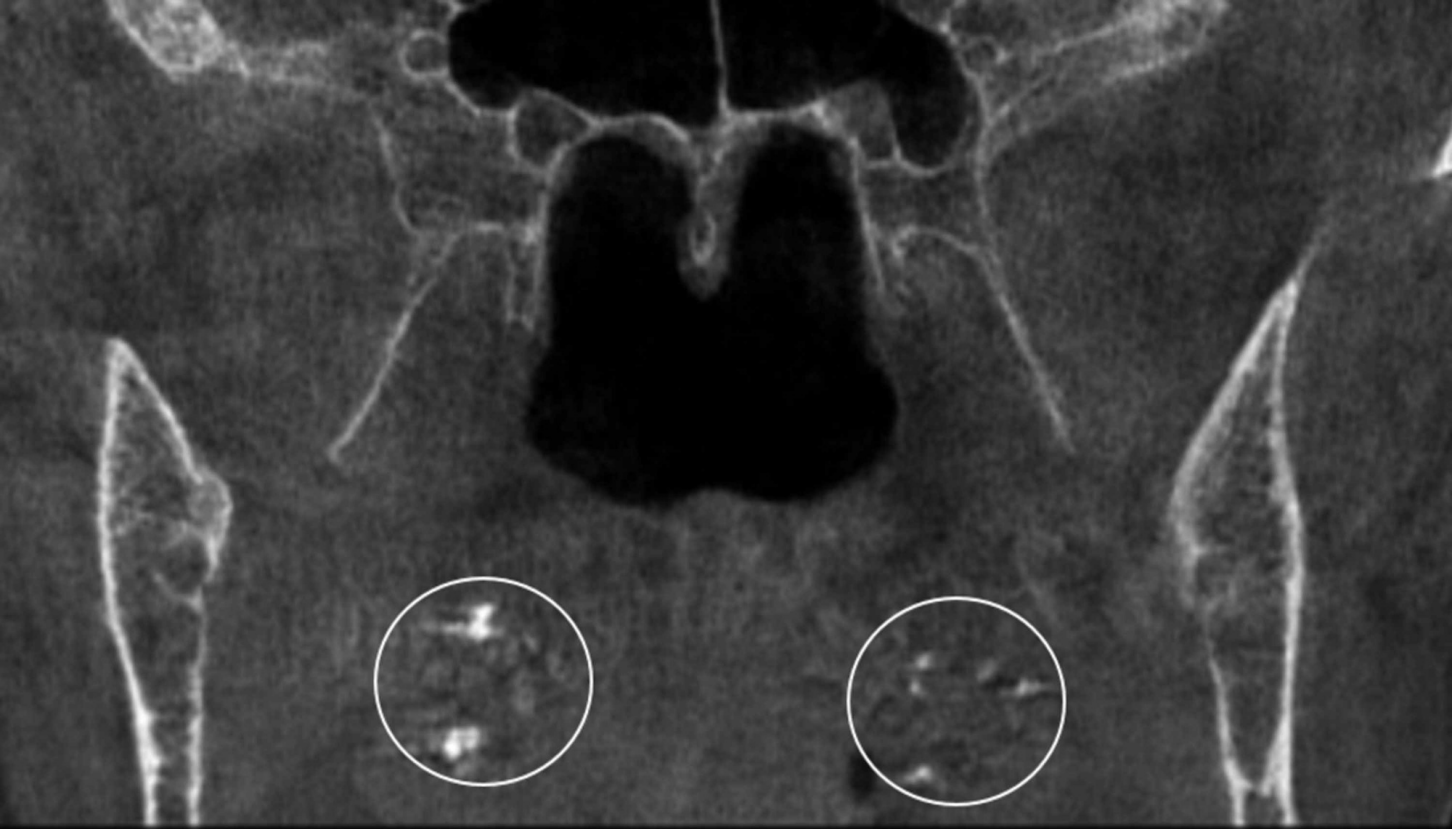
Multiple tonsilloliths in an 82-year-old female An 82-year-old female patient presenting for dental extraction. She had a history of recurrent tonsillar inflammation. A frontal cut of a CBCT, FOV: 8 cm (maxilla and mandible) shows multiple and bilateral tonsillolith calcifications (white circle). CBCT: cone-beam computed tomography; FOV: field of view
